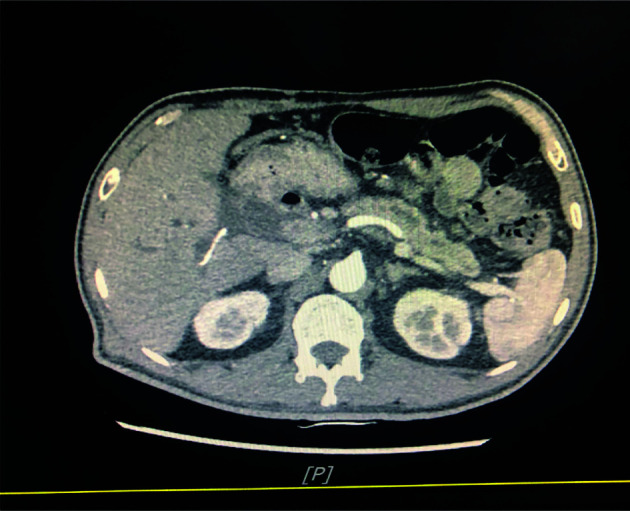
Computed tomography shows pancreatic lesion before the surgery.
